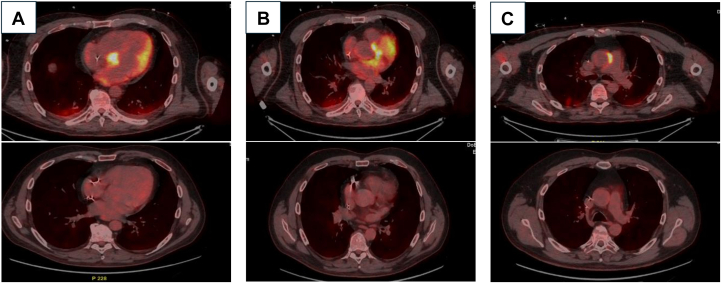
Comparison Between the Baseline ^18^F-FDG PET-CT (Top) and the Follow-Up Study Performed 1 Year After the Initiation of Immunosuppressive Therapy (Bottom) Normalization of the previously documented pathological uptake is observed at both the myocardial level (A) and the aorta (B and C).
